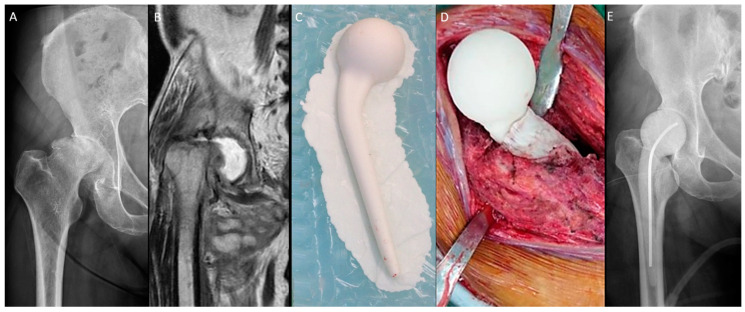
Non-medullary-sparing (n-MS) spacers. (A) Pre-operative radiograph showing cartilage destruction with the subchondral collapse of the femoral head, (B) pre-operative MRI showing diffuse fluid accumulation within the joint, (C) fabrication of the n-MS spacer using a silicone mold, (D) the hip joint with the n-MS spacer implanted, and (E) post-operative radiograph with the n-MS spacer in situ.
